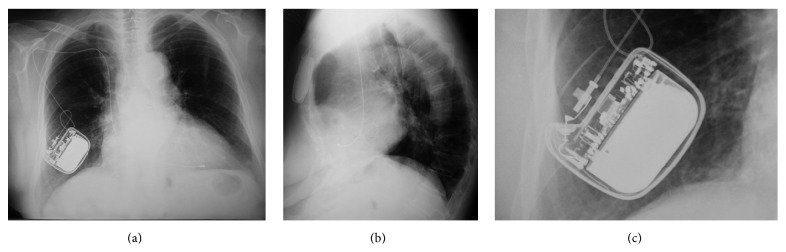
((a), (b)) Chest X-ray (May 22, 2015) of anteroposterior and lateral views. (c) Enlargement of the pulse generator figure, showing the initial generator-lead connection, without the presence of IS-1 lead connector.
